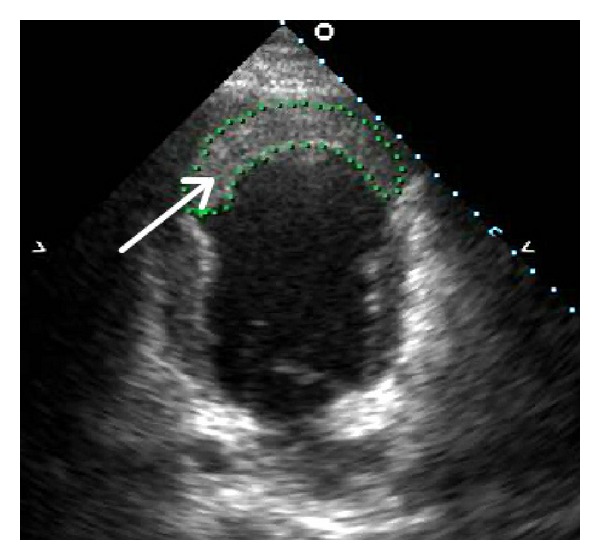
Preoperative echocardiography shows left ventricle aneurysm with the thrombus formation (arrow).
